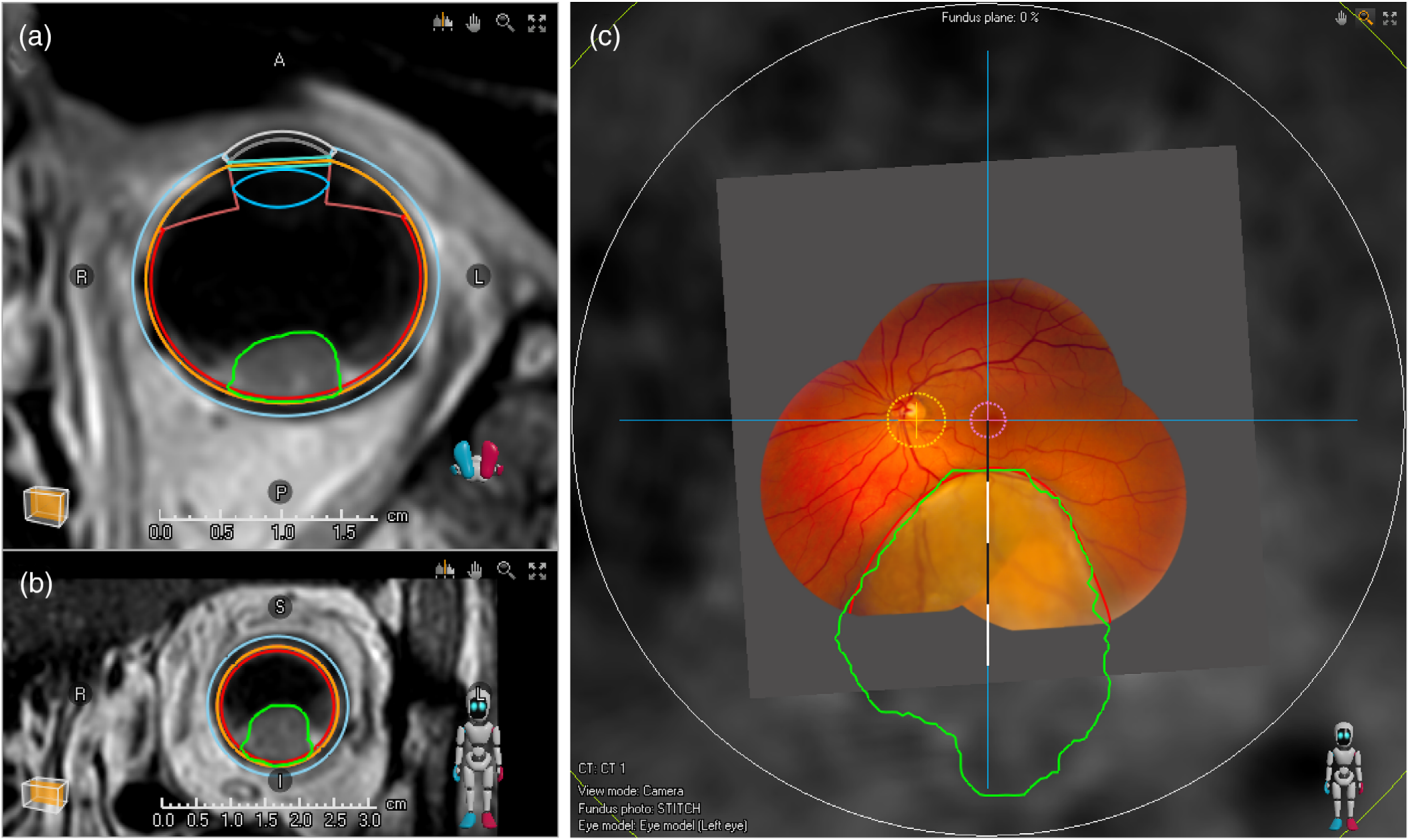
Proof of principle in RayOcular. (a,b) Transversal and coronal sections of the eye model and tumor contour (green), drawn on MRI data. (c) On the fundus projection, the MRI‐based tumor contour (green) overlaps well with the tumor in the fundus photograph (red contour). A single segment of the scale bar corresponds to 10°, so the tumor extends from 8° to 60°, which corresponds to a largest basal diameter of 13 mm. The largest difference is below 1 mm. MRI, magnetic resonance imaging.
